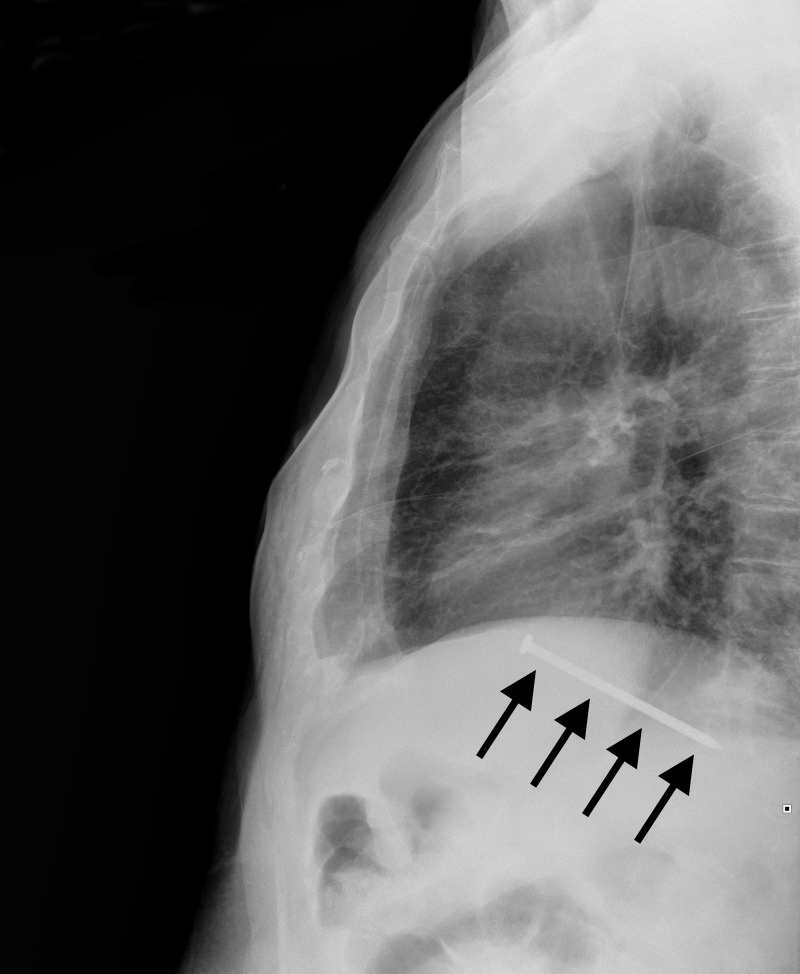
Lateral Chest X-Ray Lateral chest X-ray confirming the nail is indeed inside the thorax (arrows).
